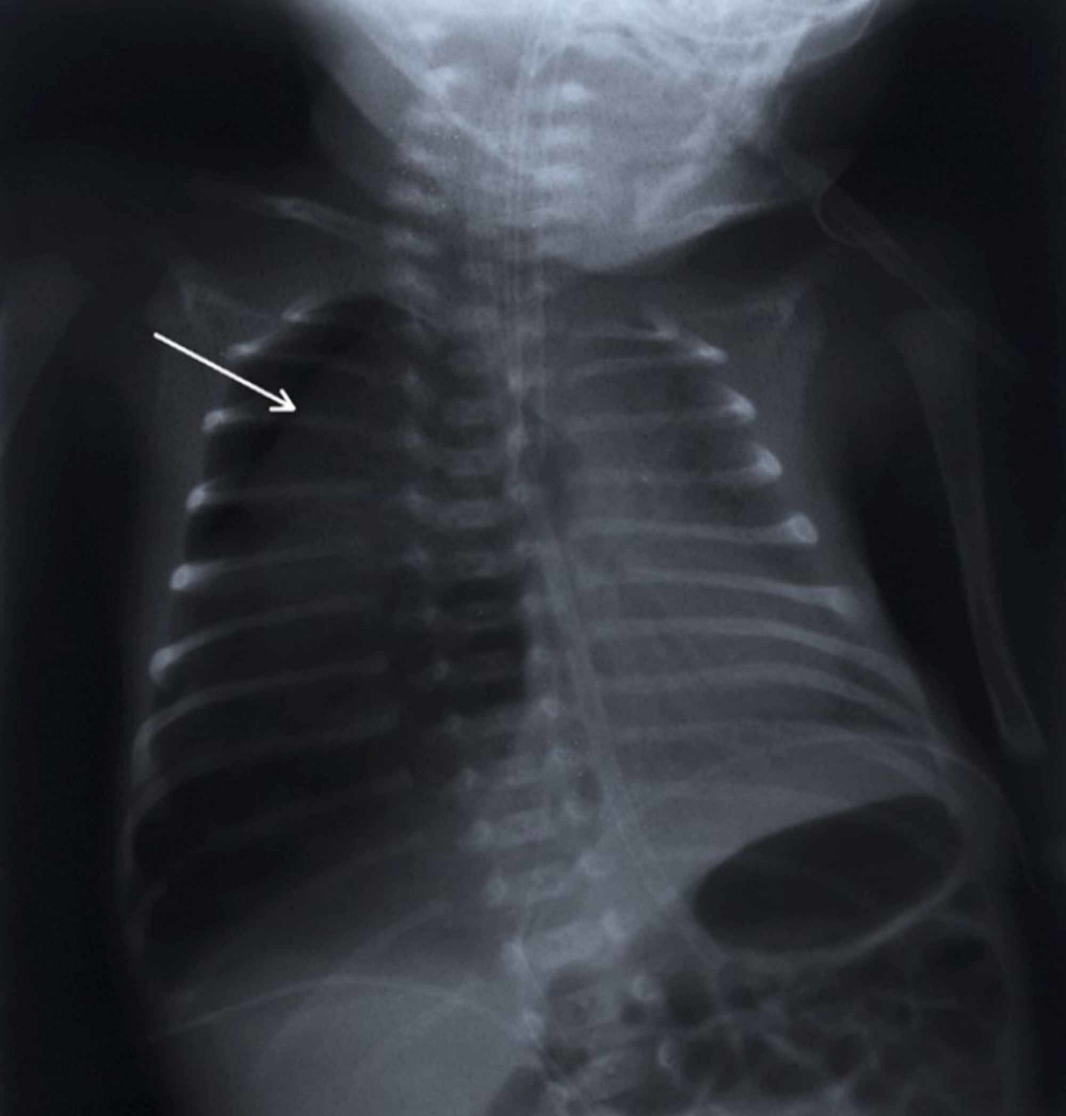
Chest radiograph, posterior-anterior view, performed after birth showing a visceral pleural line (arrow) and no lung markings in pneumothorax on the right side. Mediastinal shift to the left with compression atelectasis on the left side is present
